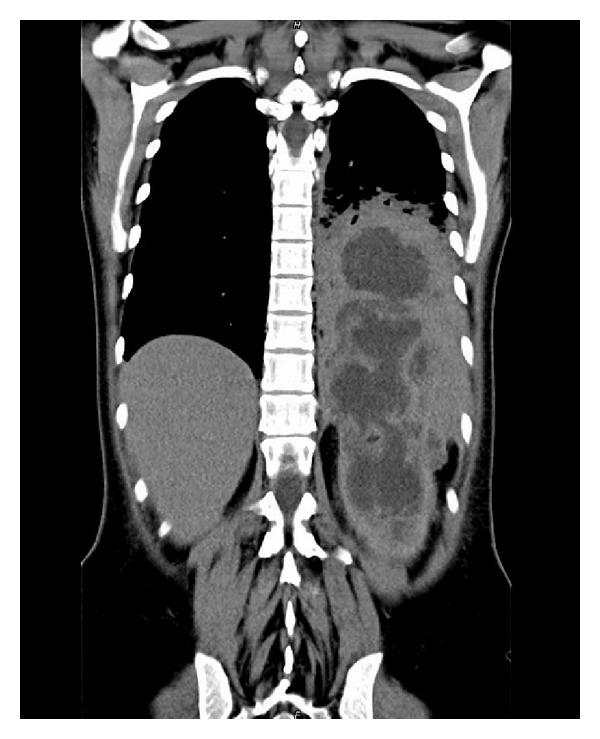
CT-scan revealing a large perinephric abscess with subphrenic extension and perforation of the diaphragm, causing a gigantic empyema which occupied the lower two thirds of the left hemithorax, totaling a 30 cm large abscess on its largest axis.
